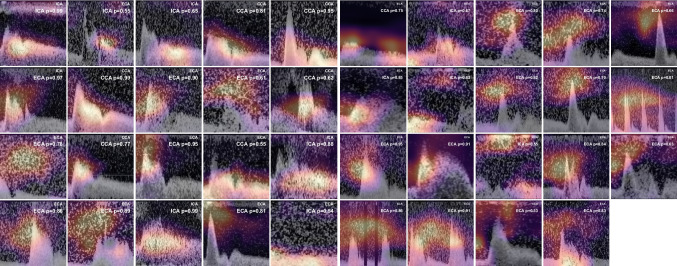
Examples of classifications on very noisy and atypical samples, such as incorrectly segmented beats, aliased Doppler, shifted peaks and multiple peaks. 29 of the 39 samples above (74%) were still correctly classified. On the top right of each image is the true label and below it is the predicted label with confidence score (p)
